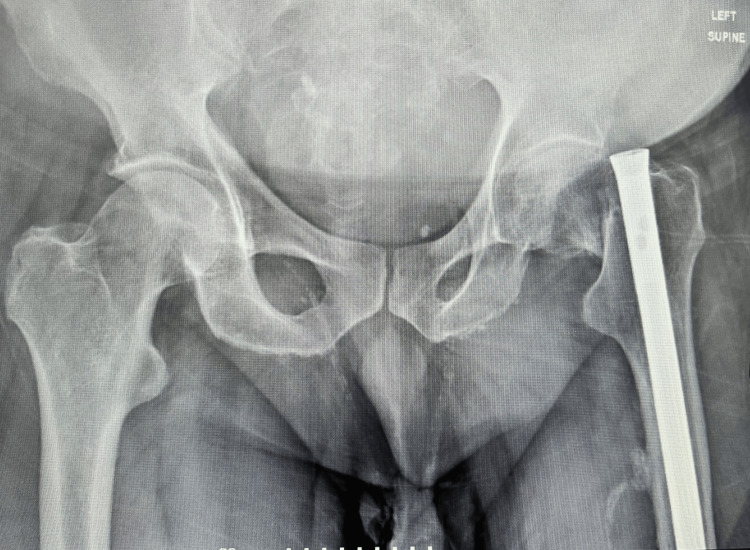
Preoperative radiograph showing a femoral neck fracture of the left hip in a patient with a retained Kuntscher nail from a previous femoral shaft fracture fixation
